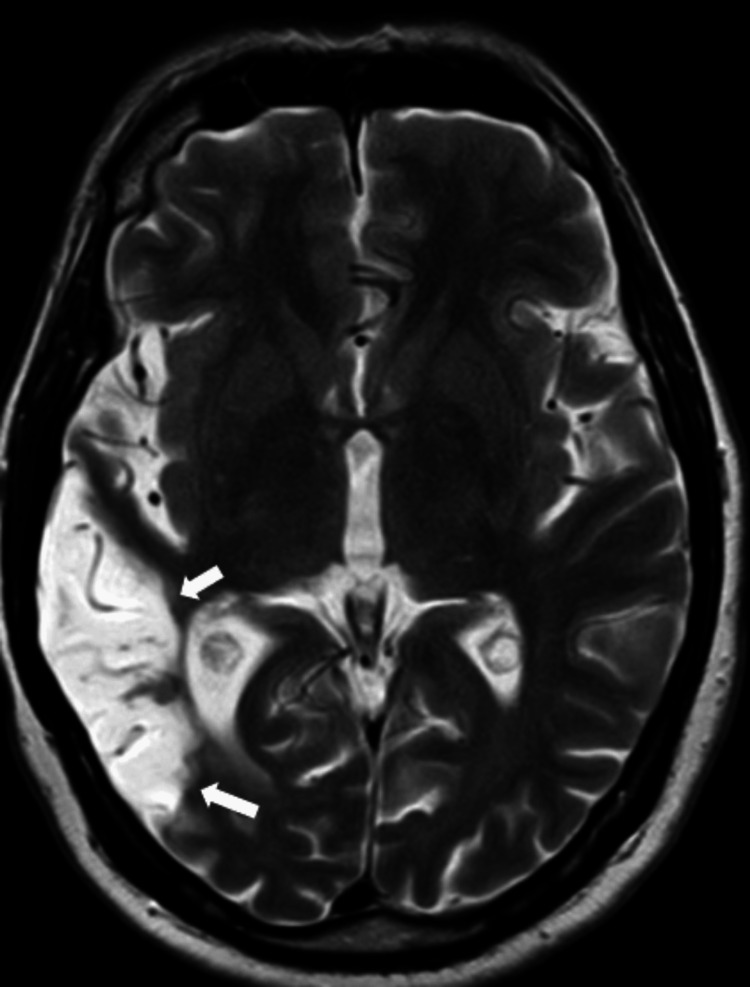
Magnetic resonance imaging (MRI) of the brain T2-weighted image (axial section) showing a hyperintense cortical lesion in the right temporal lobe
